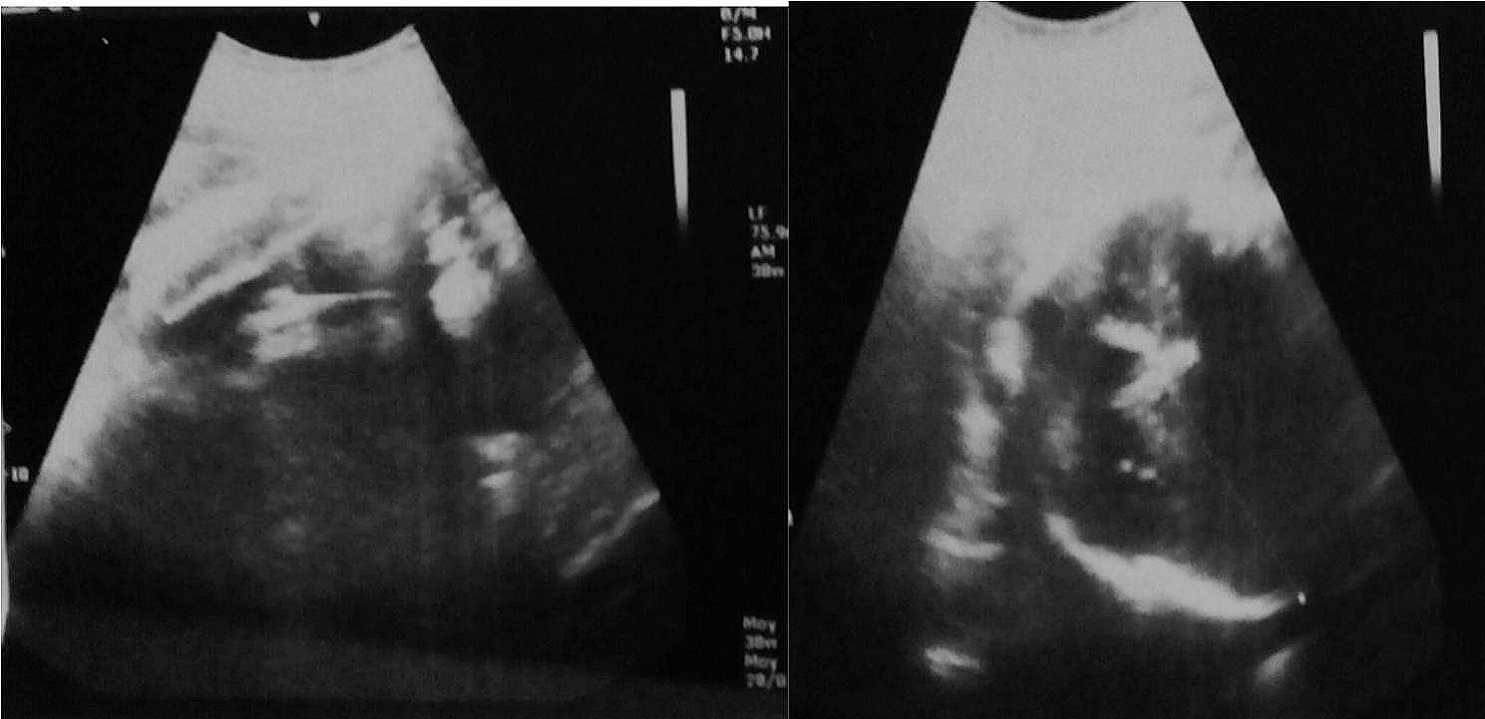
Obstetric ultrasound at 39SA. Note the absence of amniotic fluid and uterine structure circumscribing the fetal poles
